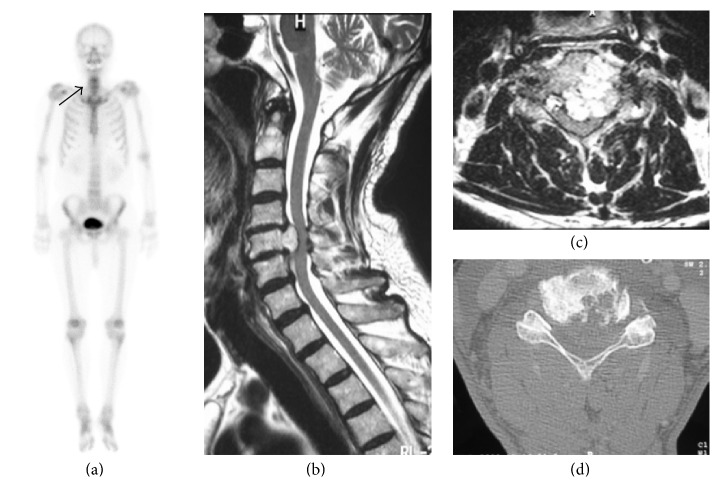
Technetium bone, magnetic resonance imaging (MRI), and computed tomography (CT) scans. (a) Technetium bone scan was useful in localizing the lesion (arrow) and no metastatic lesion was found. (b, c) The MRI scan showed a lesion of increased signal intensity on T2-weighted images in the C5 region, and the tumor is protruding into the spinal canal from the left side of the C5 vertebral body. (d) The CT scan shows the osteolytic lesion in the C5 vertebral body.
